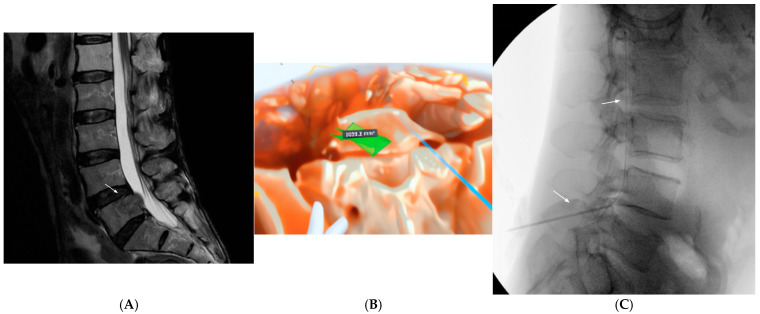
Patient 3, who did not tolerate the adverse effects of opioid therapy required for cancer pain and underwent intrathecal pump placement. (A) T2-weighted sagittal MR image demonstrating lytic lesion at L5 (white arrow). (B) Volumetric reconstruction of patient imaging, with polygons used to demarcate the tumor and planning of the path of entry. (C) Successful intrathecal pump placement using an L3/4 right paramedian approach. White arrows delineate the path of the intrathecal catheter.
